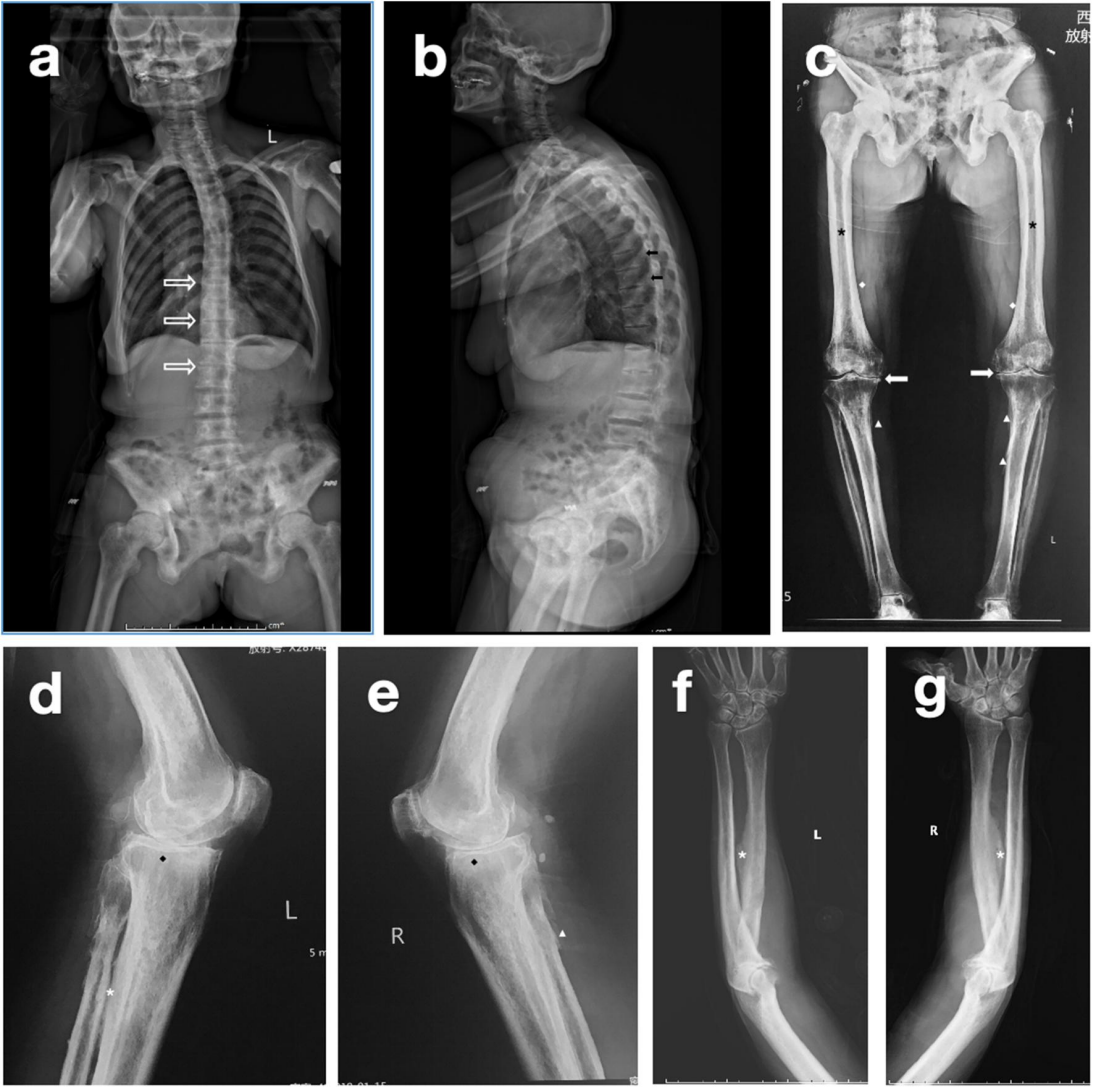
Preoperative radiographic appearance: (a) anteroposterior and (b) lateral views of the spine show typical bamboo-shaped changes (hollow white arrow) and calcification of the ligamentum flavum (black arrow) in the thoracic and upper lumbar vertebrae. (c) Full-length anteroposterior view of the left (d) and right knees. (e) The lateral view shows a varus deformity, a narrowed medial joint space (solid white arrow) with increased bone density, a fused trabecular bone (black rhombus), a thickened cortical bone (white rhombus), a narrowed marrow cavity (black star), ossification of ligaments (white triangle), and interosseous membranes (white star). (f) Anteroposterior and (g) lateral views of the forearm show ossification of interosseous membranes (white star).
